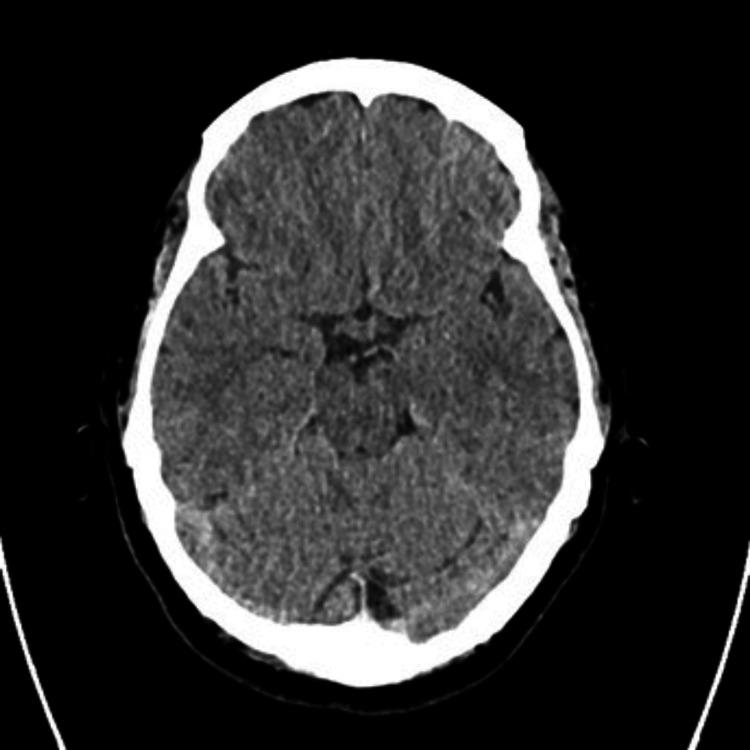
Normal noncontrast CT scan of the brain This figure shows a CT scan of the brain performed to rule out SAH, which was normal. SAH: Subarachnoid hemorrhage.
